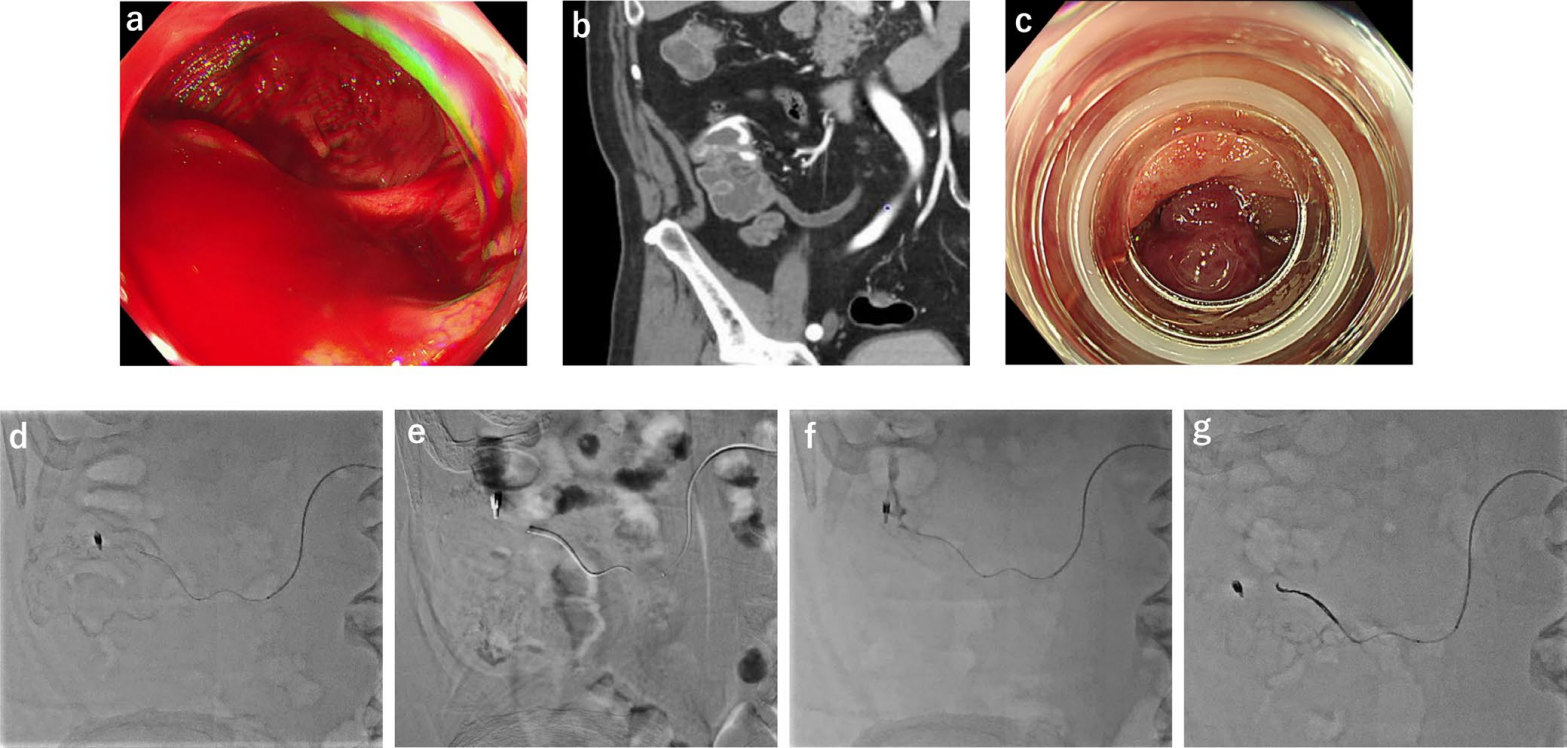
(a) Active bleeding began in the ascending colon, making it difficult to maintain the visual field. (b) Contrast‐enhanced CT revealed extravasation in the ascending colon. (c) Endoscopic band ligation was performed on the diverticulum with an exposed vessel. (d) Initial iodinated angiography revealed no extravasation due to spontaneous hemostasis. (e) CO2 was injected into the artery responsible for bleeding for further assessment. (f) A repeat iodinated contrast study revealed extravasation from the ascending colonic diverticulum. (g) Coil embolization was finally performed on the artery responsible for bleeding.
